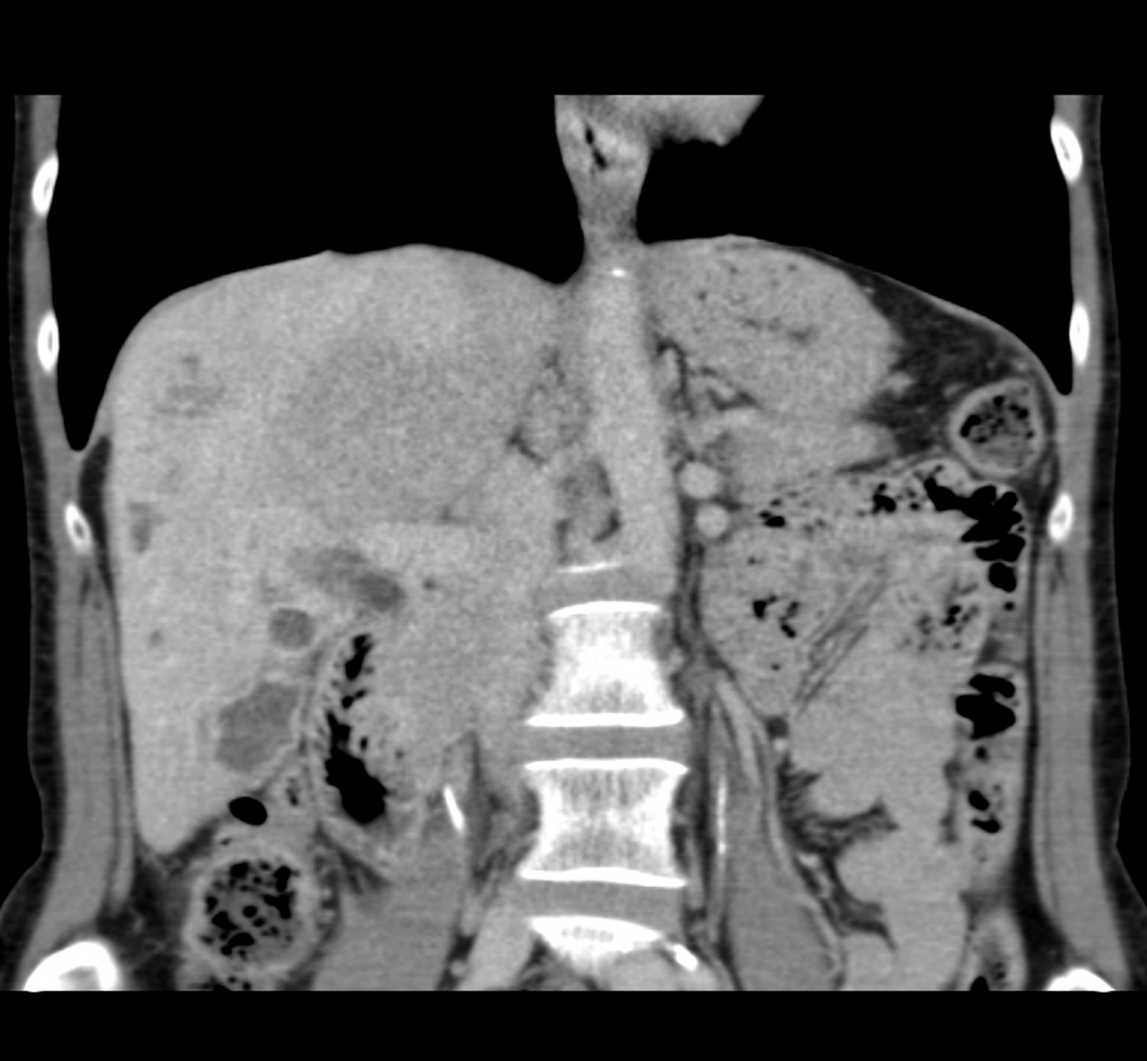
Representative coronal slice from CT obtained three months after second SIRT procedure
